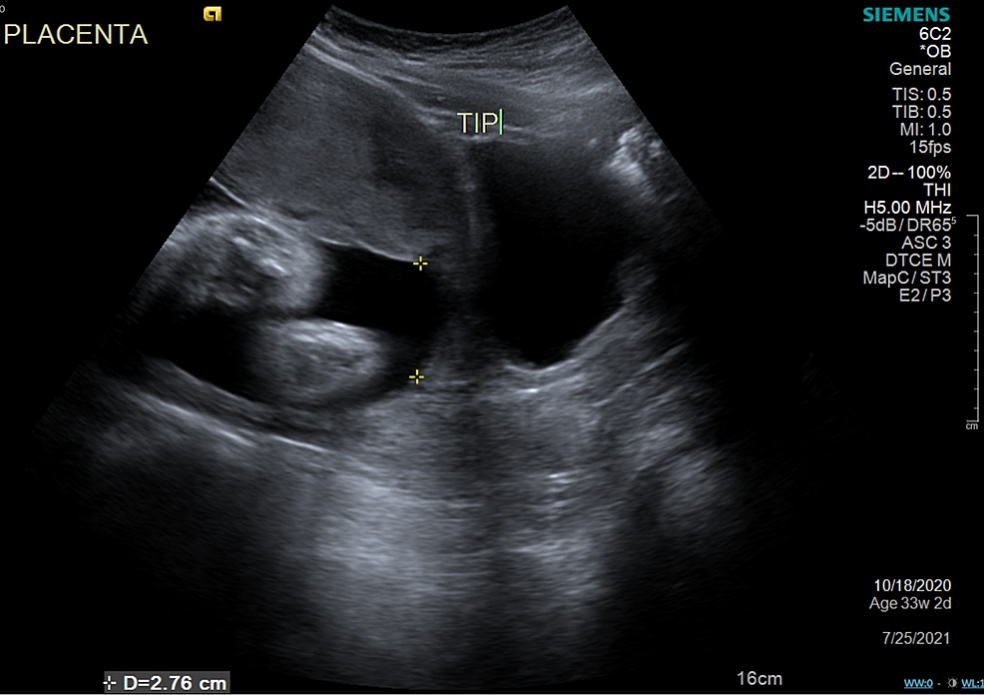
Transabdominal ultrasound measuring the placenta edge to be 2.76 cm from the internal cervical os.
